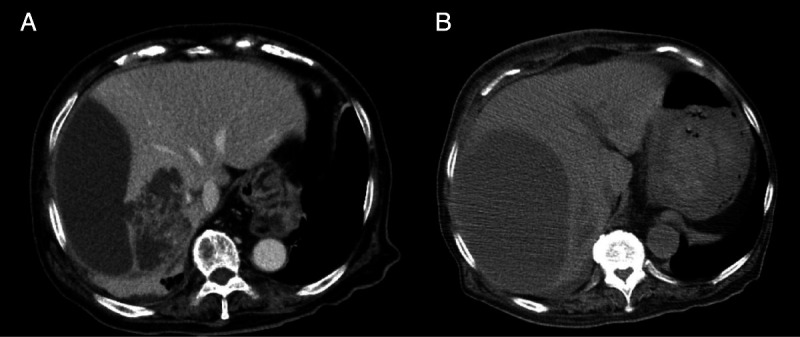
Computed tomography revealed multiple abscesses in the right lobe of the liver (A). The abscess in the right liver lobe was enlarged (B).
